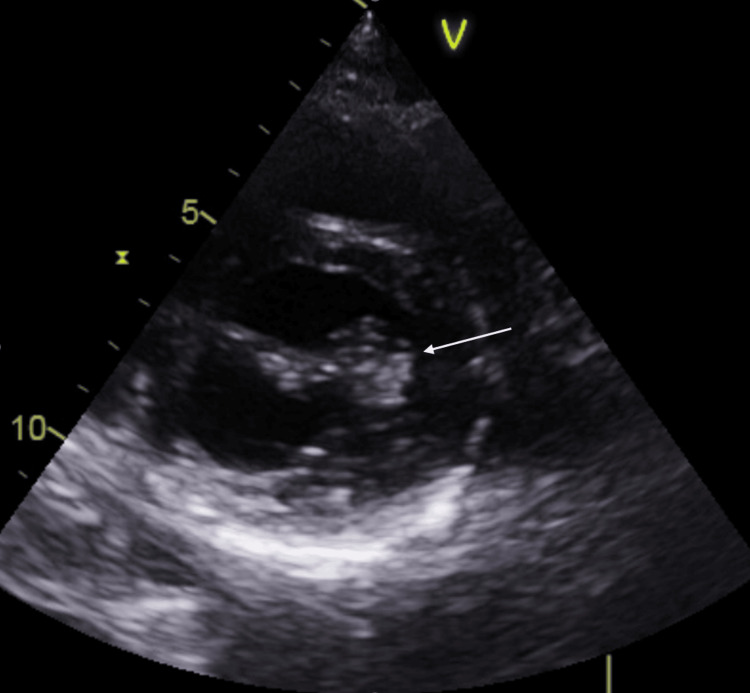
Echocardiogram demonstrating mitral valve mass.
